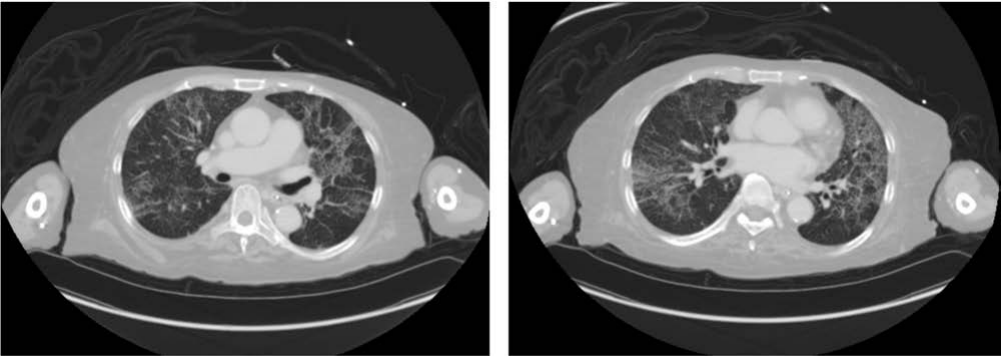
Initial chest X-ray and computed tomographic findings. multifocal patchy consolidations and ground-glass opacity (GGO) in both lungs.
